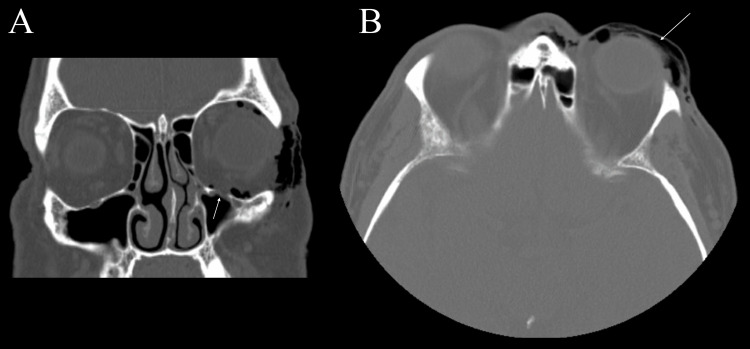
Maxillofacial CT scans without contrast A. Coronal view highlighting a small 5 mm anteromedial orbital floor defect (white arrow) with orbital emphysema and no orbital content herniation or depression fracture. B. Axial view highlighting extensive orbital emphysema (white arrow).
